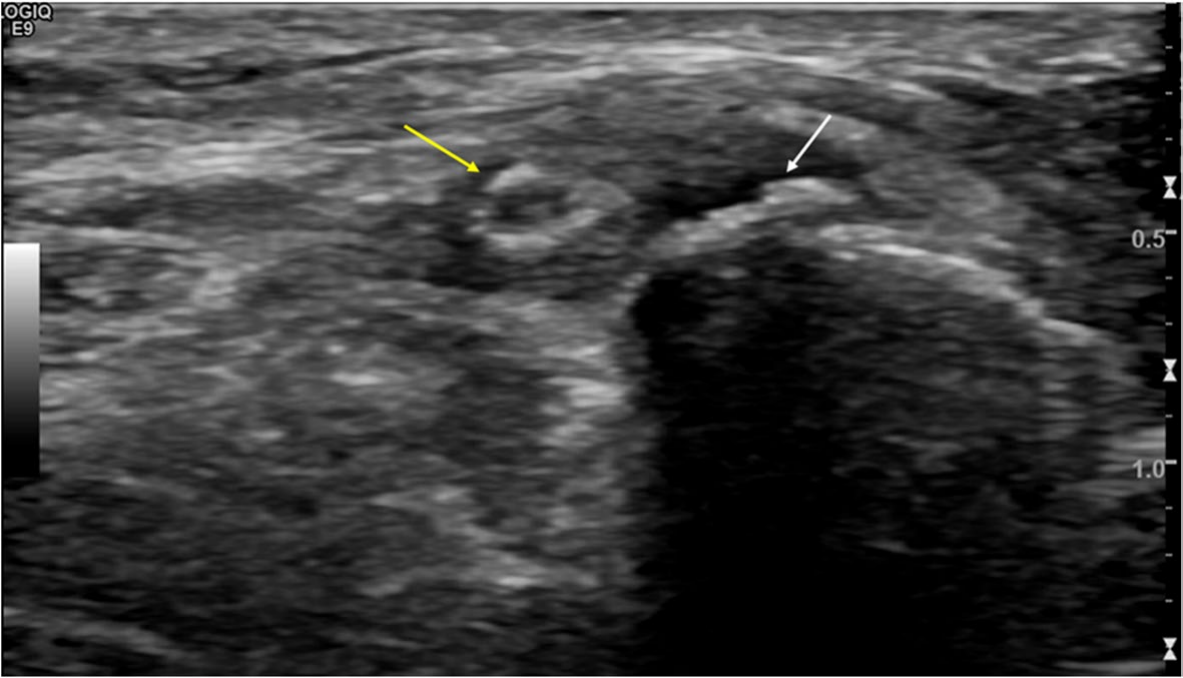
45-year-old male with enthesopathy at the anterior superior iliac spine (ASIS). Ultrasound demonstrates a traction spur arising from the ASIS (white arrow) with thickening of the inguinal ligament and lateral femoral cutaneous nerve (yellow arrow)
